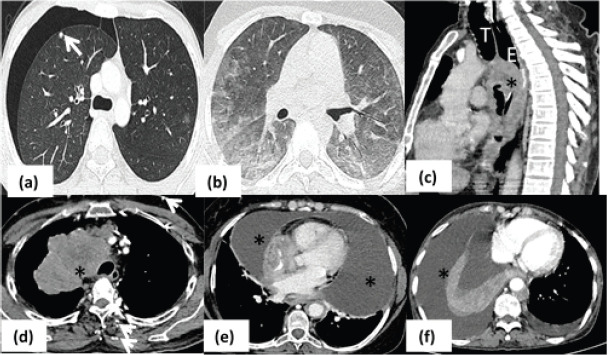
Pulmonary complications. (a): Axial HRCT image in a patient with osteosarcoma showing presence of subpleural metastatic nodule (arrow) in right upper lobe with right sided pneumothorax. (b): Axial HRCT image showing peripheral and lower lobe predominant ground glass opacities in bilateral lung fields in a case of Hodgkin’s lymphoma on chemotherapy s/o bleomycin toxicity. (c): Sagittal CT image of patient with esophageal cancer (asterisk) demonstrating airway invasion anteriorly (arrow). (d): Conglomerate mediastinal mass (asterisk) proven as lymphoma is seen to cause complete obliteration of SVC with multiple chest wall collaterals (arrows) – SVC syndrome. (e): Axial mediastinal window of a patient with leukemia who had massive pericardial effusion (asterisk) causing respiratory distress. (f): Axial CT iamge of lung cancer patient with gross right sided pleural effusion (asterisk) causing collpae of underlying lung. (T- trachea; E- esophagus).
